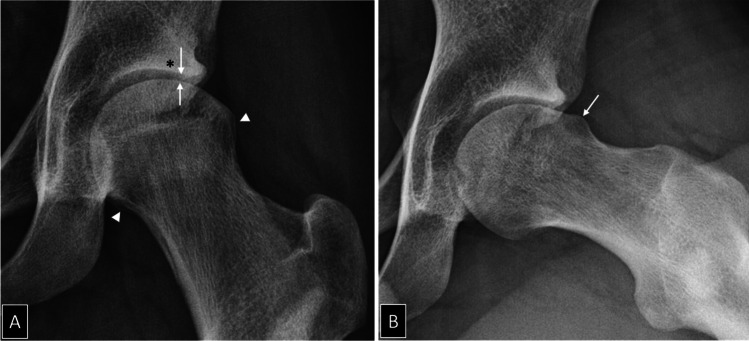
A 21-year-old man with moderate left hip pain and osteoarthritis secondary to femoro-acetabular impingement. A AP radiograph demonstrates lateral joint space narrowing (arrows), subchondral sclerosis of the acetabular roof (asterisk) and femoral head osteophytes (arrowheads). B The 45° Dunn lateral view demonstrates Cam deformity at the head-neck junction (arrow)
